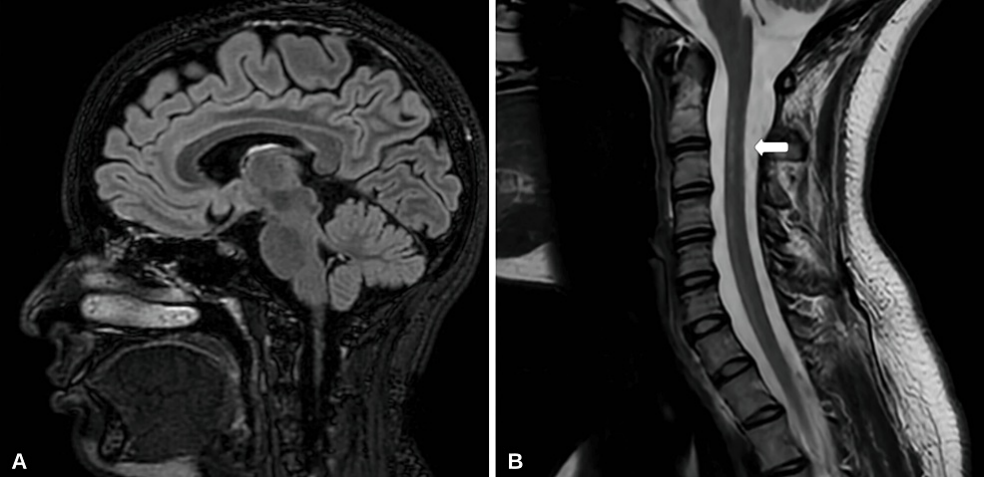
Repeat MRI of the brain and cervical spine (A) MRI of the brain T2/FLAIR sagittal sequence reveals resolution of previous splenium lesion on the corpus callosum. (B) MRI of the cervical spine T2/FLAIR sagittal sequence depicts improvement in hyperintense lesions (arrow). MRI: magnetic resonance imaging, FLAIR: fluid-attenuated inversion recovery
